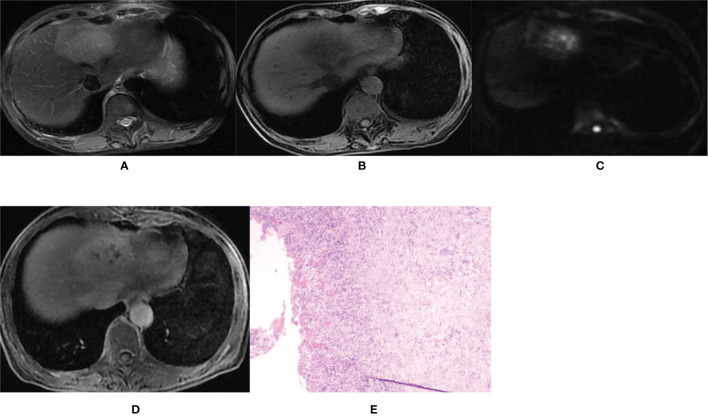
Imaging of hepatic abscess. A 60-year-old female patient was admitted to our hospital mainly due to a fever for 4 days with the white blood cell count of 9.38×10^9^/L and the percentage of neutrophils of 83.1%. (A, B) T2WI and T1WI showed a round mass shadow in the left lobe with a clear boundary, slightly longer T1 and slightly longer T2 signal. (C) The DWI image showed multiple high spotted signals in the center, and the central signal was higher than the peripheral signal. (D) On the hepatobiliary-phase enhanced imaging, the lesion showed a slightly high peripheral signal and patchy low signal in the center. The │CNR│ was 17.17 in the peripheral region but 13.21 in the central. The visibility score was 3. (E) Pathological sections showed fibrocystic wall tissue with acute and chronic inflammatory cell infiltration, but no clear lining epithelium. Some small vessels were dilated and congested, which was consistent with the wall of a liver abscess. The pathologic diagnosis was a hepatic abscess.
